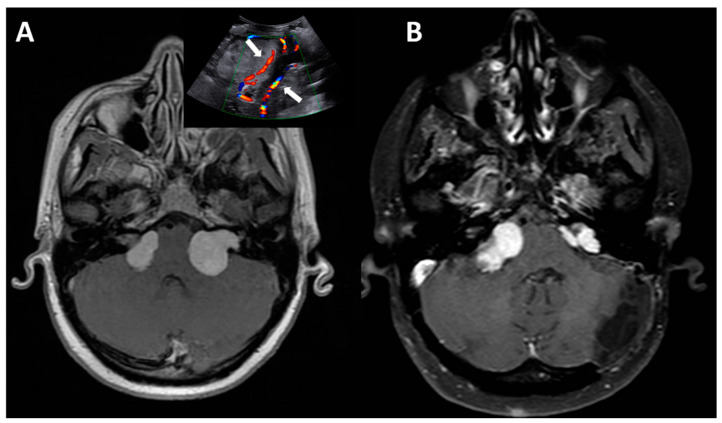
Example of tumor arising directly form the CPA. (A) Axial gadolinium MRI showing a bilateral CPA schwannoma in a 14-year-old girl with NF-2. In the box, IUOS demonstrate the relationship of the left tumor with the vertebral arteries (arrows); (B) Postop MRI performed 3 months after surgery showing the subtotal removal of the left tumor and the progression of the right one.
